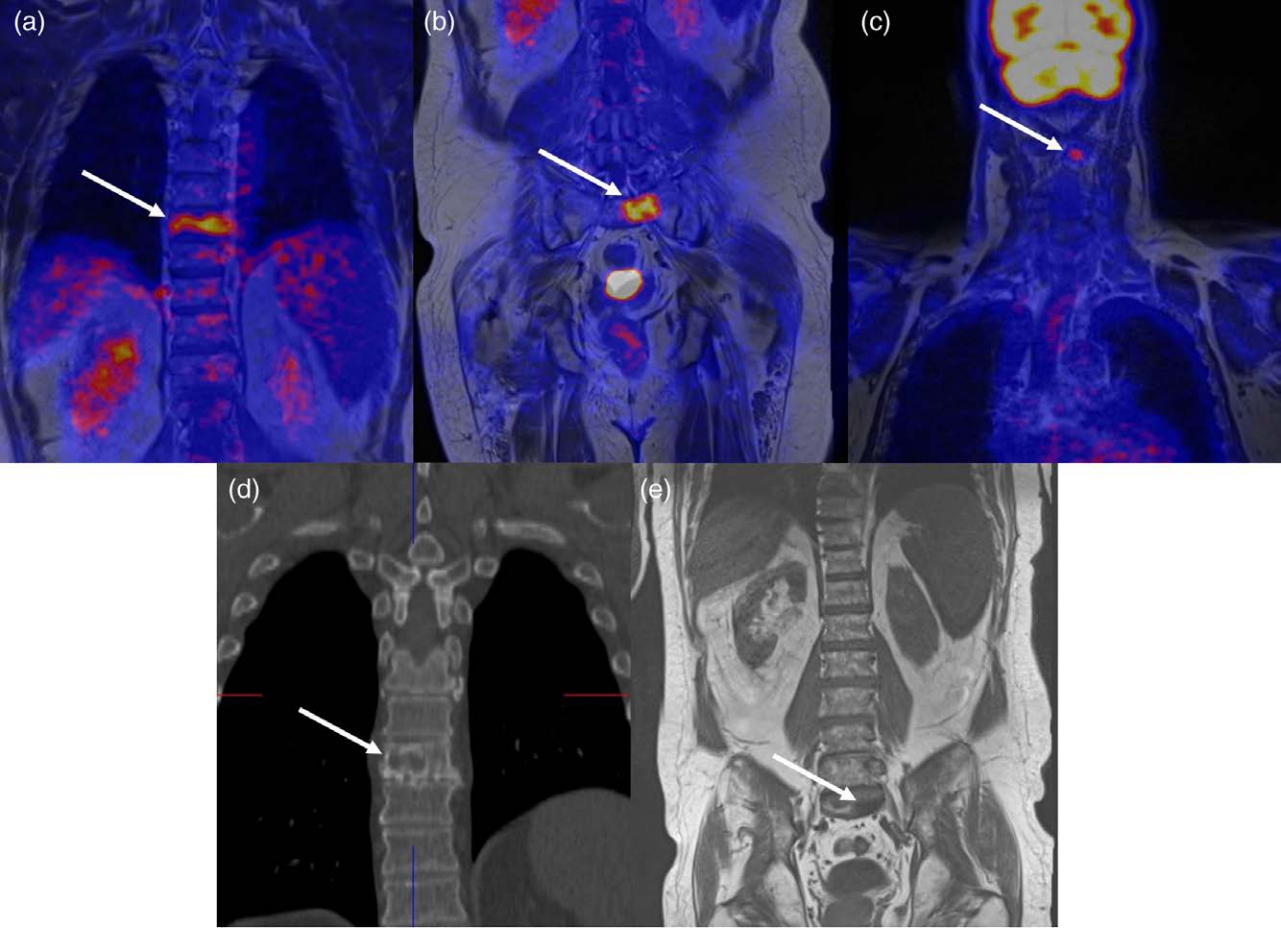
PET/MRI showing three hypermetabolic bone metastases (arrows) in the dorsal spine (a), sacrum (b), and cervical spine (c); (d) CT coronal reconstruction showing the osteolytic lesion (arrow) in the dorsal spine; (e) coronal T1 MR scan showing the bone metastasis (arrow) in the sacrum. The bone metastasis in the cervical spine was not identified by thoracic CT plus abdominal MRI, as it was out of the standard field of view.
